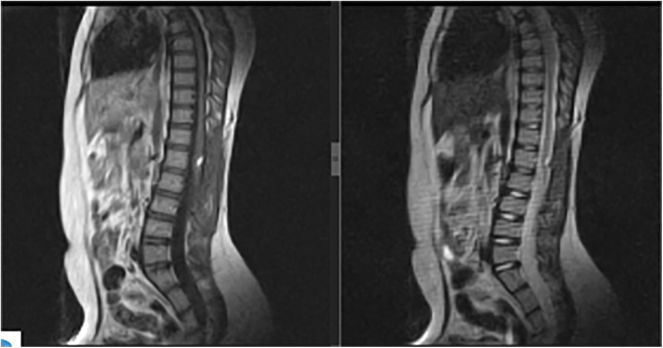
Postoperative MRI shows reduction of the lipoma with a slightly remained lesion.
